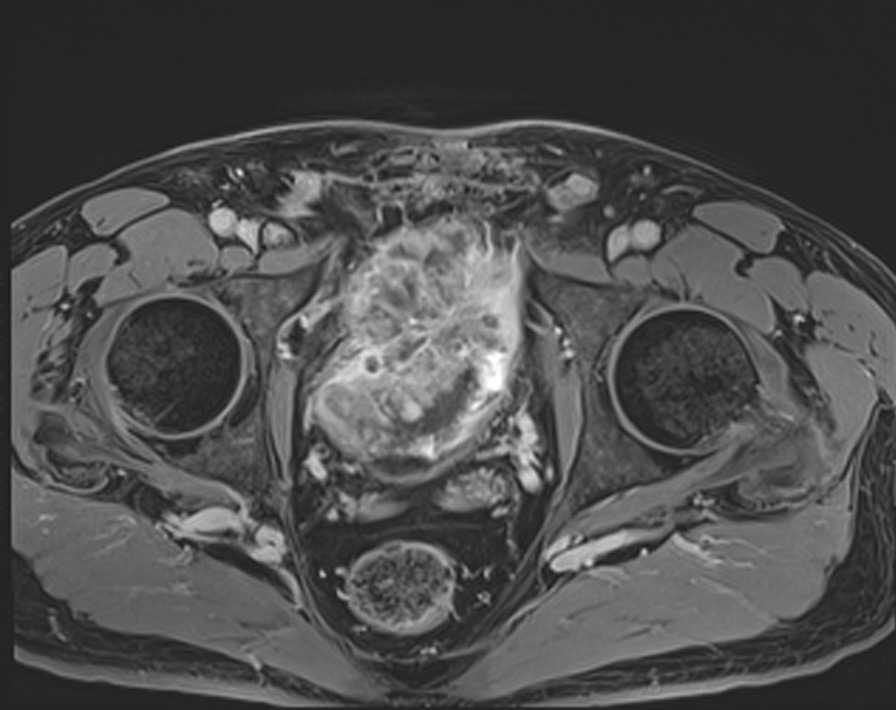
Pelvic MRI T1-weighted axial image showing anterior extrusion of the tumor on the right without pelvic side wall involvement
